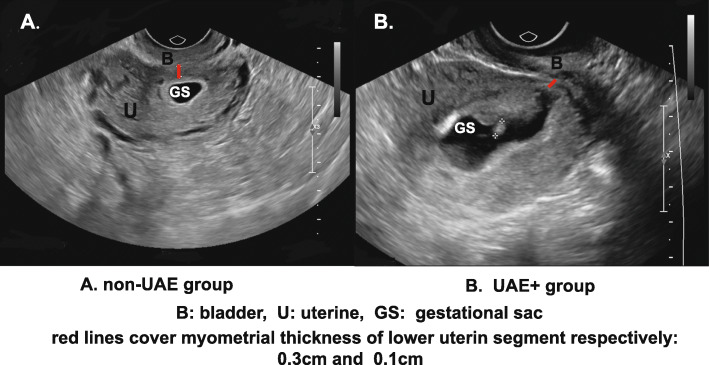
Representative ultrasonographic images from the two groups
